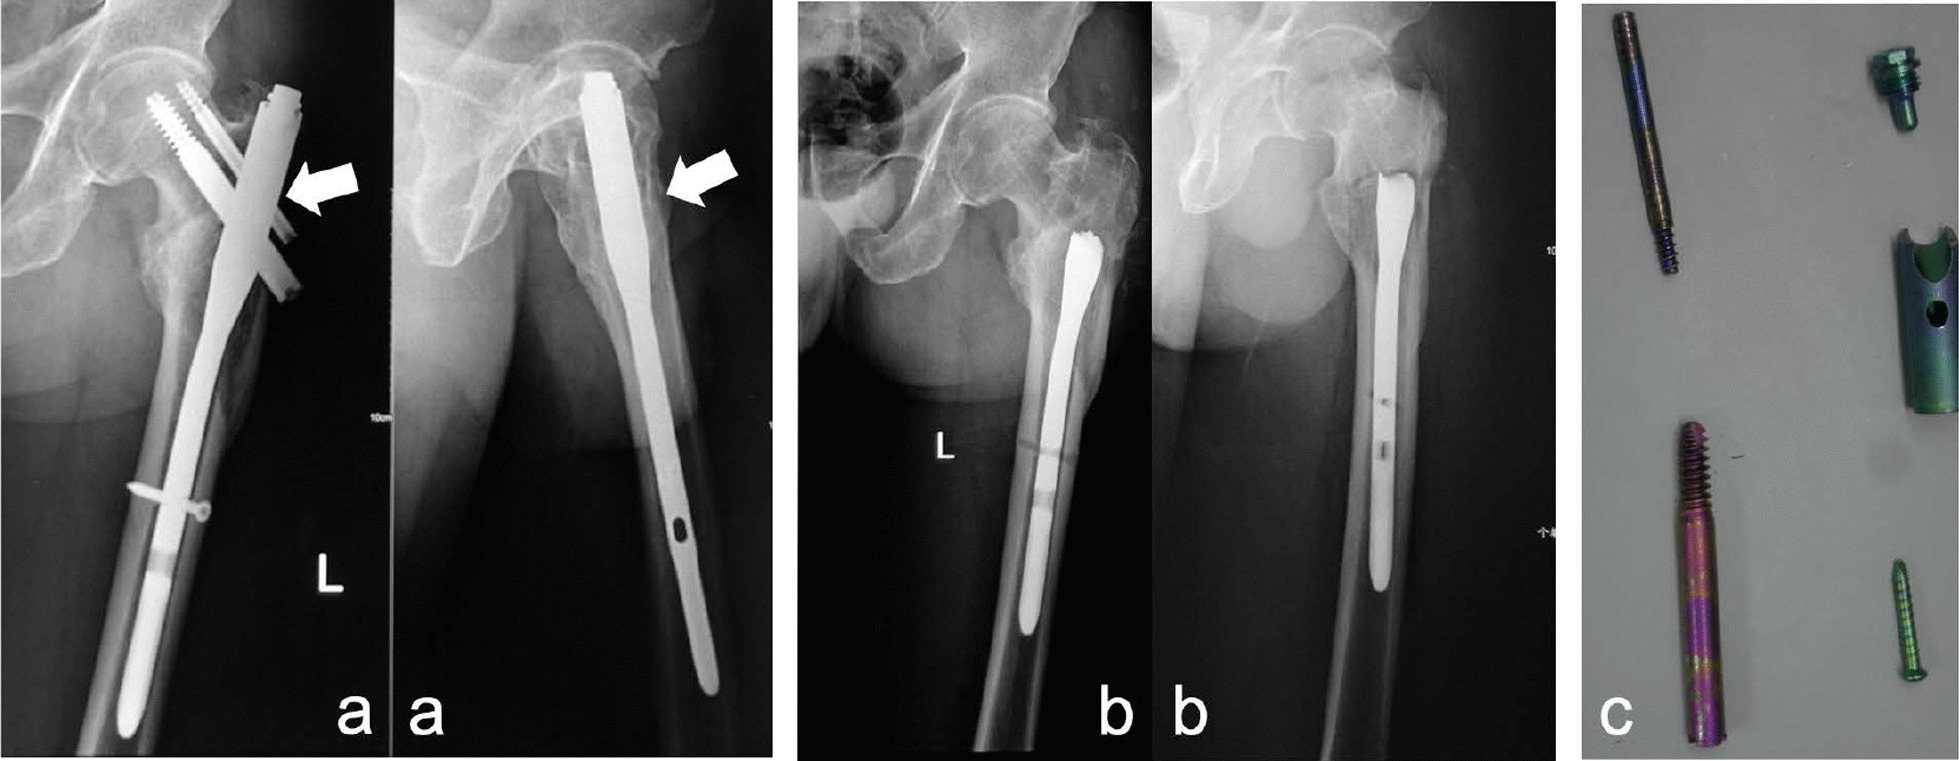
A 79-year-old male with a 31 A3 fracture was treated with a short PFN, and the fracture was healed. The patient complained of progressive discomfort in the lateral hip 4 years after the initial operation and decided to undergo removal of the PFN 3 years later. a No signs of nail breakage were observed on the X-rays before implant removal. b, c Nail breakage was found during the operation, and only part of it was removed to avoid iatrogenic injury
